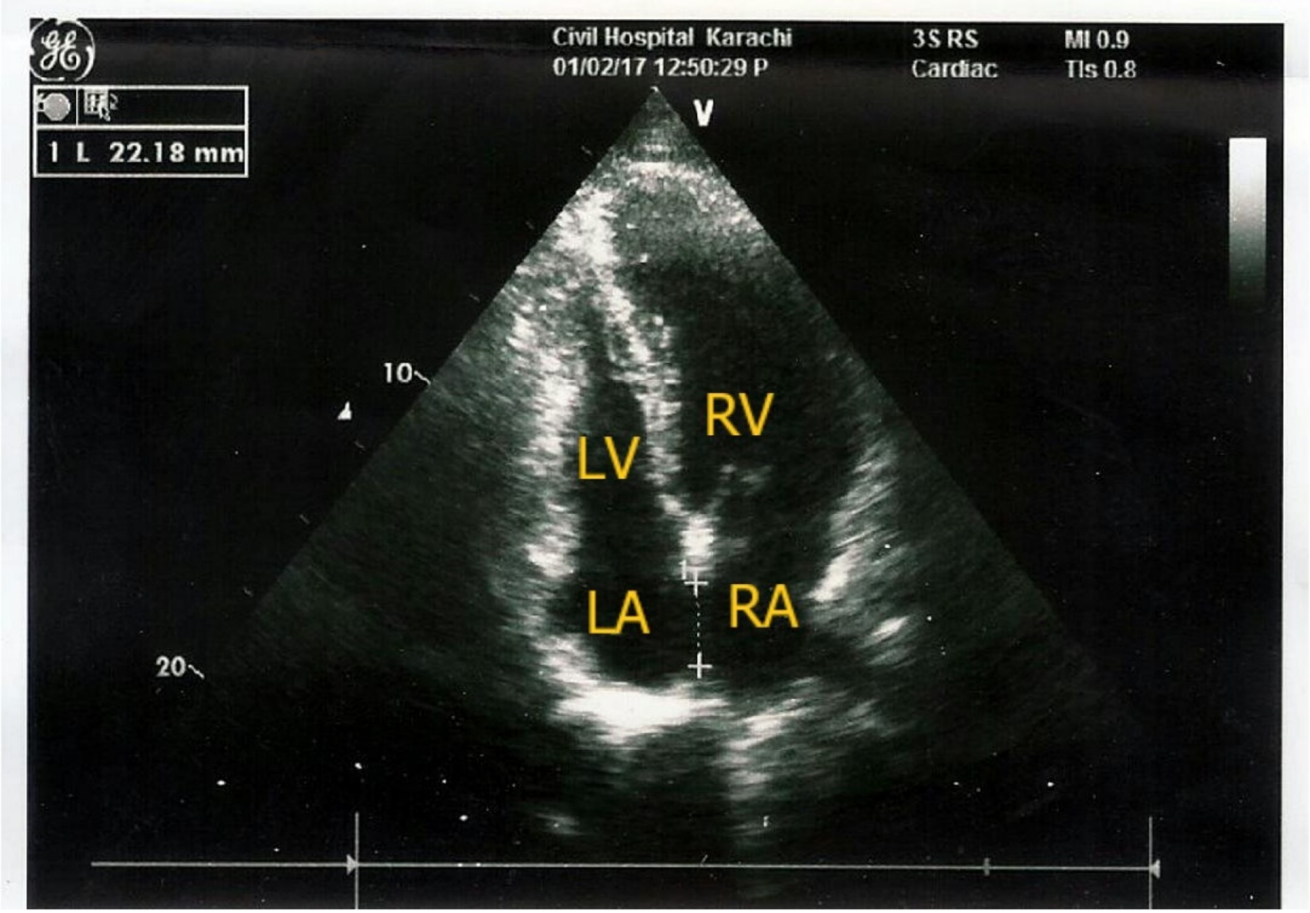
Apical four chamber view showing a large atrial septal defect (ASD)
